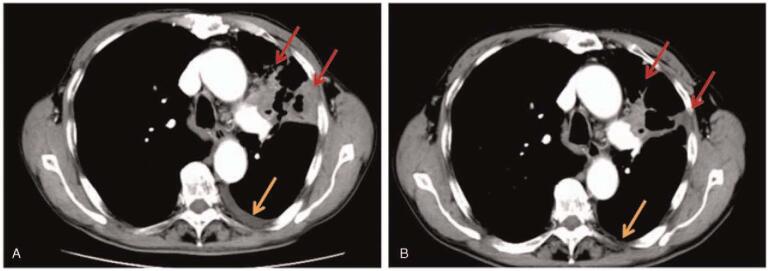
Chest CT images obtained after 2 course (A) and 4 course (B) of treatment with sintilimab combined with endostar and nab-paclitaxel. The red arrows indicated lung masses and the yellow arrows indicated pleural effusion. CT = computed tomography.
